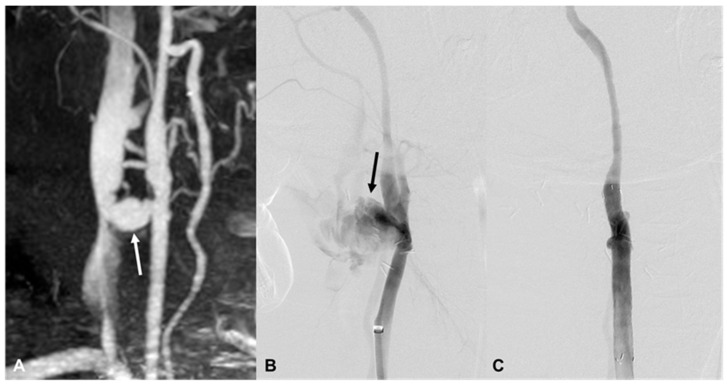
A 47-year-old man (patient 12) with a stab injury. (A) CT angiography shows a fistula between the right distal common carotid artery (CCA) and the internal jugular vein (arrow). (B) DSA shows massive contrast extravasation from the right distal CCA (arrow). (C) The lesion has disappeared after placement of the covered stent.
